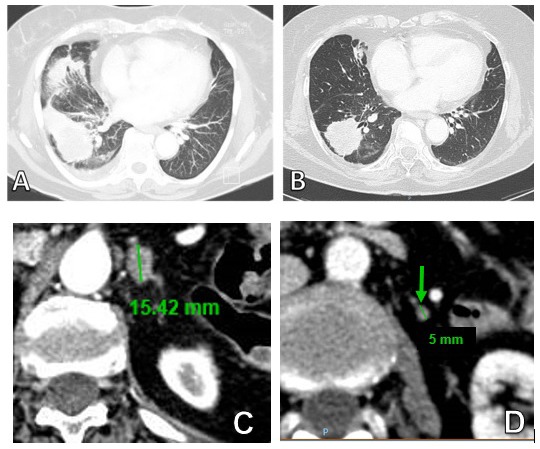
Thoracic CT scan of primary lesion (A) at baseline and (B) after 60 day of treatment with Afatinib. (C) Adrenal metastasis at baseline (D) and response to treatment after 60 days.
